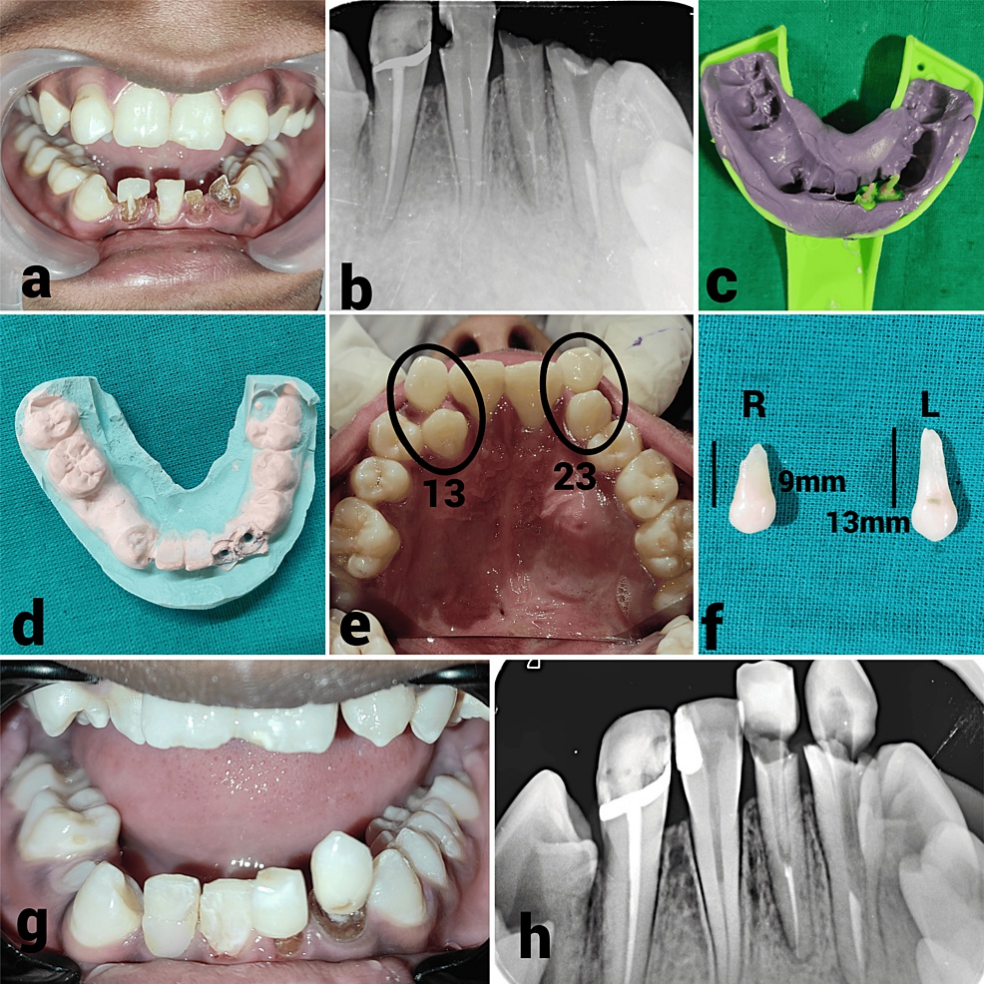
Sequential stages of endodontic intervention of lower anterior teeth (a) Preoperative photograph revealed grossly decayed teeth 32, 33, and 41; (b) Obturation of teeth 32, 33, and 41 done using gutta-percha and coated with AHPlus sealer (Dentsply Sirona, York, USA); (c) Impression of the post space of teeth 32 and 33 made with a custom-made self-cure post and medium-bodied elastomeric impression material; (d) Impression was poured with die stone to create a model, which served as a guide during the fabrication of the dentin post; (e) Extraction of both maxillary supernumerary teeth (left and right) was performed; (f) Extracted teeth were thoroughly cleaned and autoclaved at 121°C for 15 minutes; (g) Each tooth was trimmed circumferentially along the root portion to match the length of the respective root canal of teeth 32 and 33; (h) The model served as a reference for orienting the shape, length, and thickness of the dentin post, which was radiographically verified.
